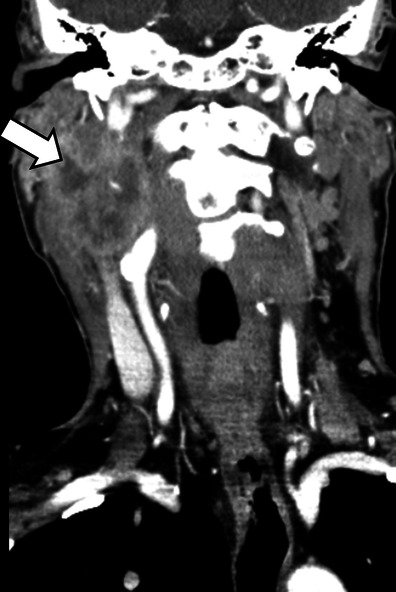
Lymph node metastasis in the neck from oropharyngeal squamous cell carcinoma.*A 66-year-old man with a history of smoking and alcohol abuse presenting with a swelling on the right side of the neck and right VCP. Coronal contrast enhanced CT images showed a large lymph node conglomerate at level IIb (*arrow*) on the right side of the neck, with necrosis, extra nodal spread and extensive involvement of the right carotid space explaining the right VCP in this patient
